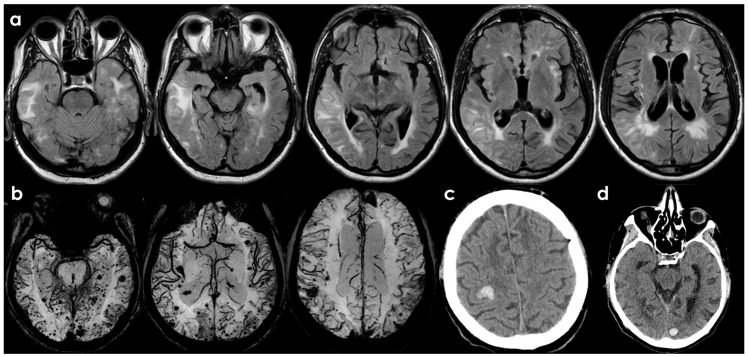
Brain MRI of a patient (male, 72 years old, past history of arterial hypertension) admitted for the occurrence of decreased alertness due to non-convulsive status epilepticus. In panel (a), axial Fluid Attenuated Inversion Recovery (FLAIR) slices in ascending sequences show the multilobar subcortical white matter hyperintensity with finger-like projections into the axis of cortical giri, with a tumefactive effect, simultaneous cortical swelling and sulcal effacement in the involved regions and sulcal hyperintensities. The involved regions are anterior temporal lobe on both sides, the right lateral temporal lobe extended to the lateral temporo-parietal transition and the posterior parietal lobe, and the right fronto-polar region. In panel (b), axial Susceptibility Weighted Imaging (SWI) images, reconstructed by using minimum Intensity Projections/MultiPlanar Reconstruction (minIP/MPR) protocol, showing an extensive burden of CMBs with few hypointense lesions > 1 cm (macrohemorrhages). In panels (c,d), non-contrast computed tomography (NCCT) of the brain at two sequential time points in the follow-up, showing acute ICH.
